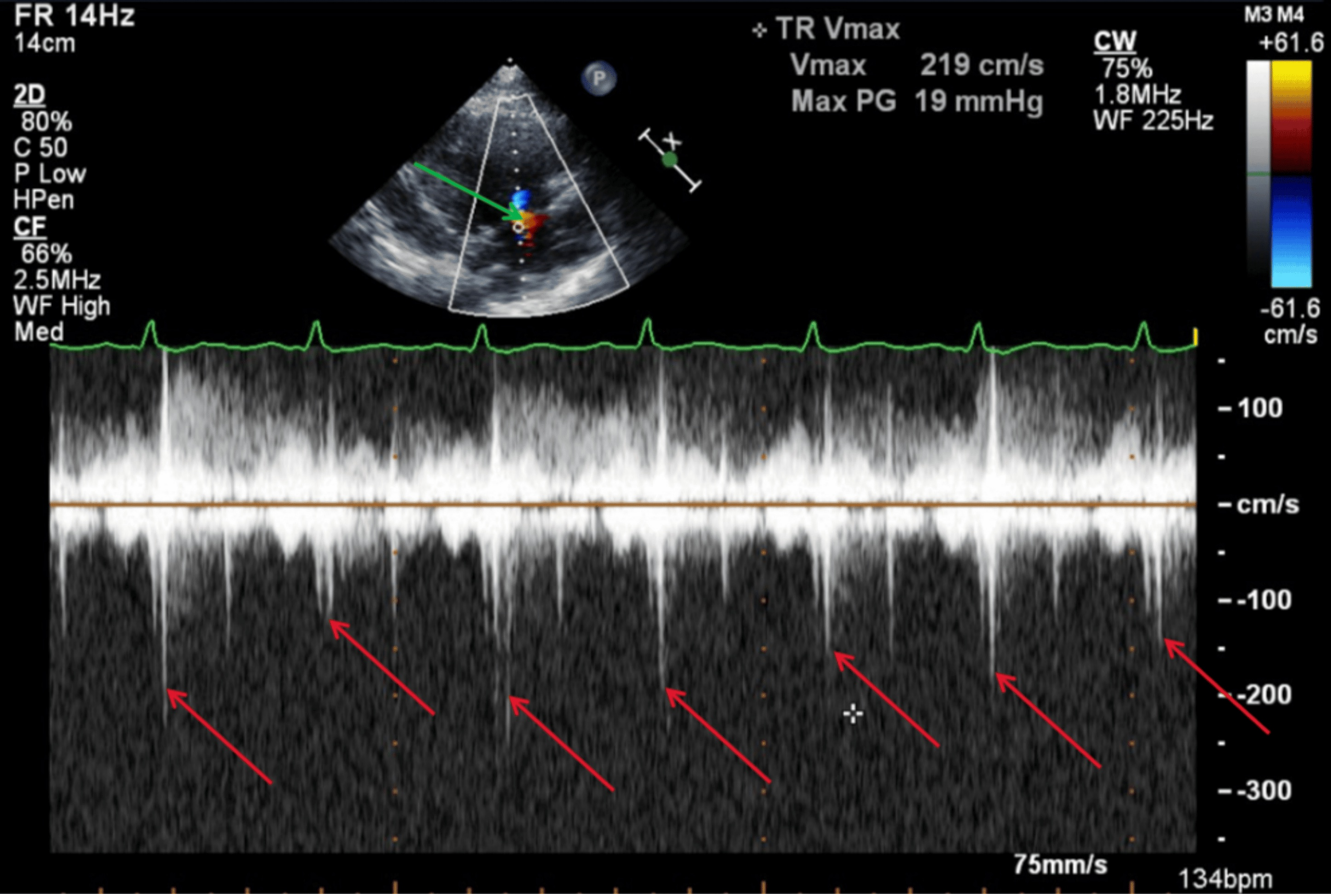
TTE parasternal short-axis view with color Doppler and M-mode during systole. The green arrow shows findings of mild tricuspid valve regurgitation with color Doppler. The red arrows show mild tricuspid valve regurgitation with various velocities with each systole. TTE: transthoracic echocardiography
